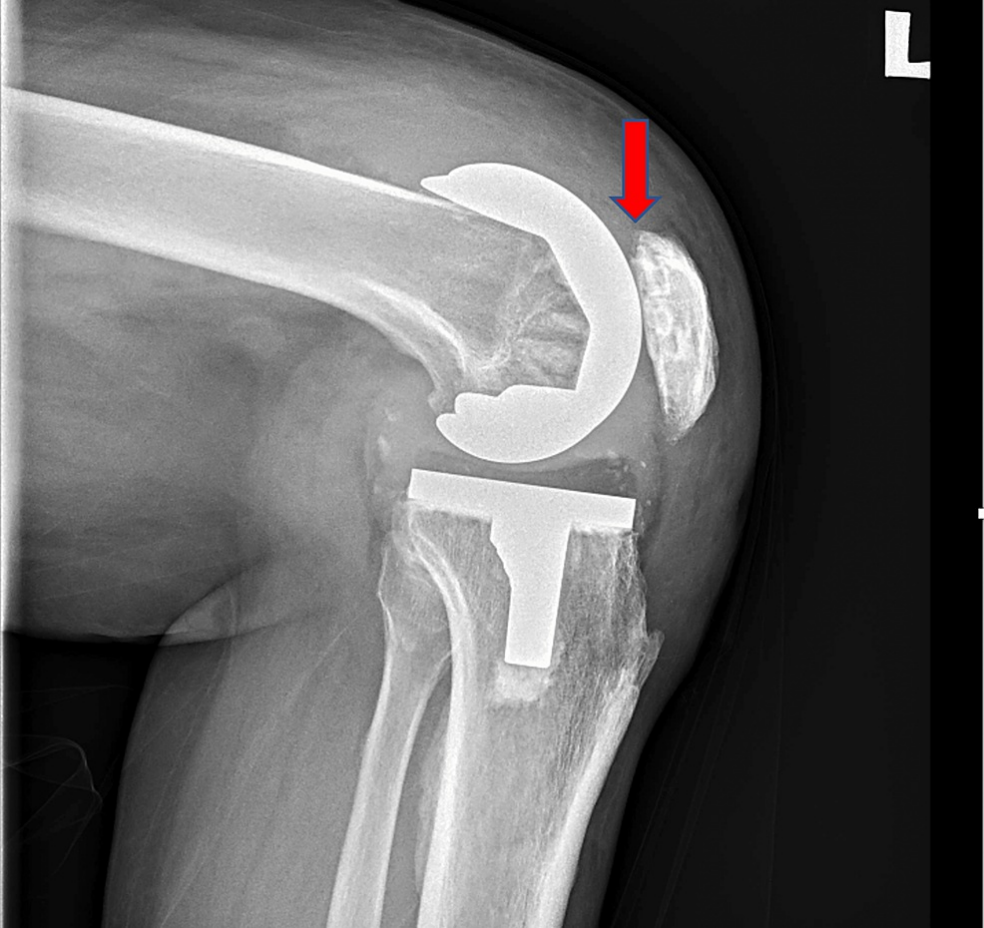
Lateral radiograph of the knee showing well-seated implants and patella re-resurfacing (arrow)
